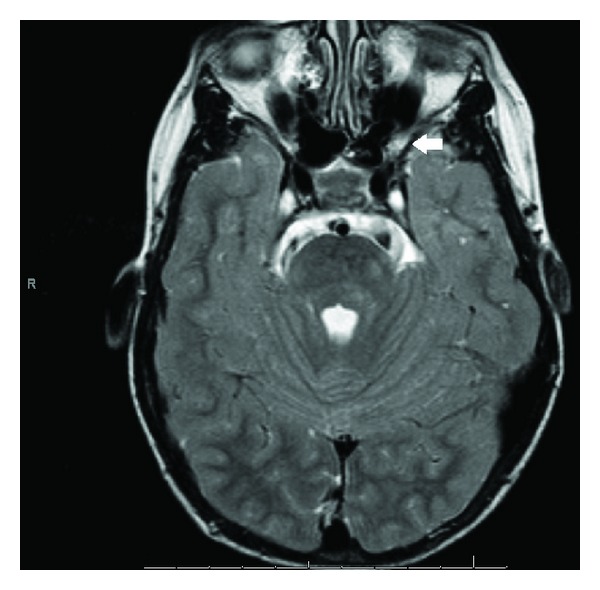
Brain MRI: axial section (T2) showing optic nerve glioma.
